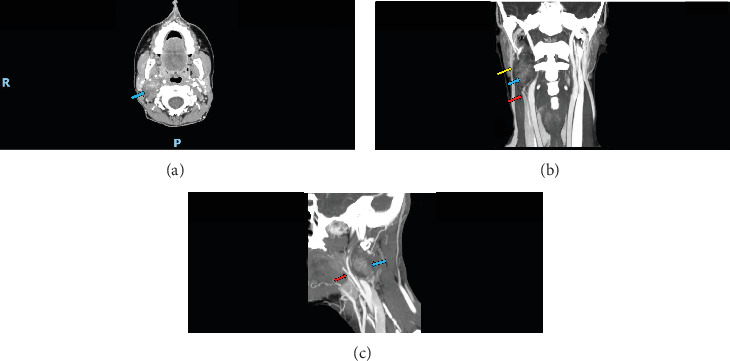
Angio CT scan. (A) Axial view, (B) coronal view, and (C) sagittal view. Blue arrow—tumor; Red arrow—carotid artery compression; Yellow arrow—vertebral artery compression.
